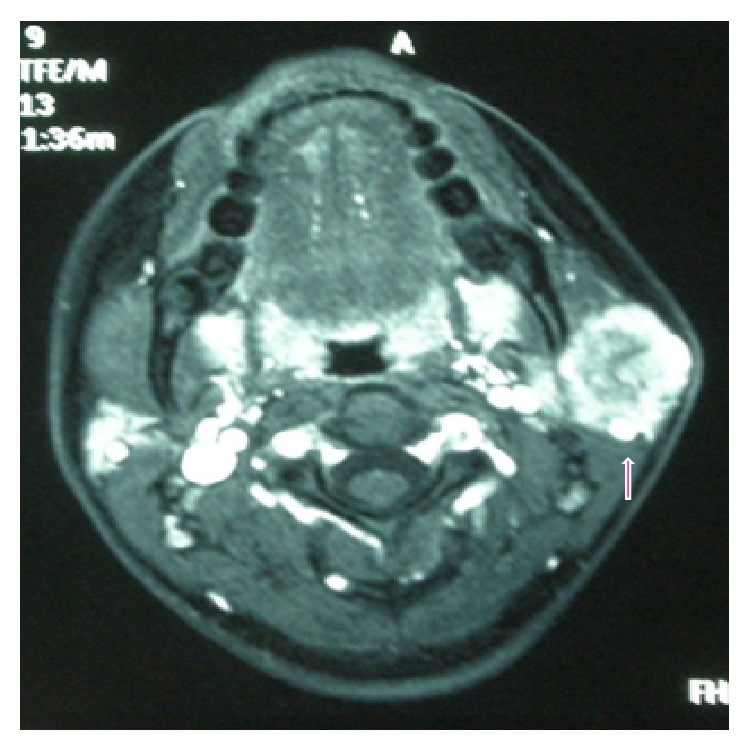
Contrast-enhanced computed tomography images of the tumor. A 2.8 cm sized tumor was present at the left parotid gland. The tumor was relatively circumscribed and contained a low-density area inside.
